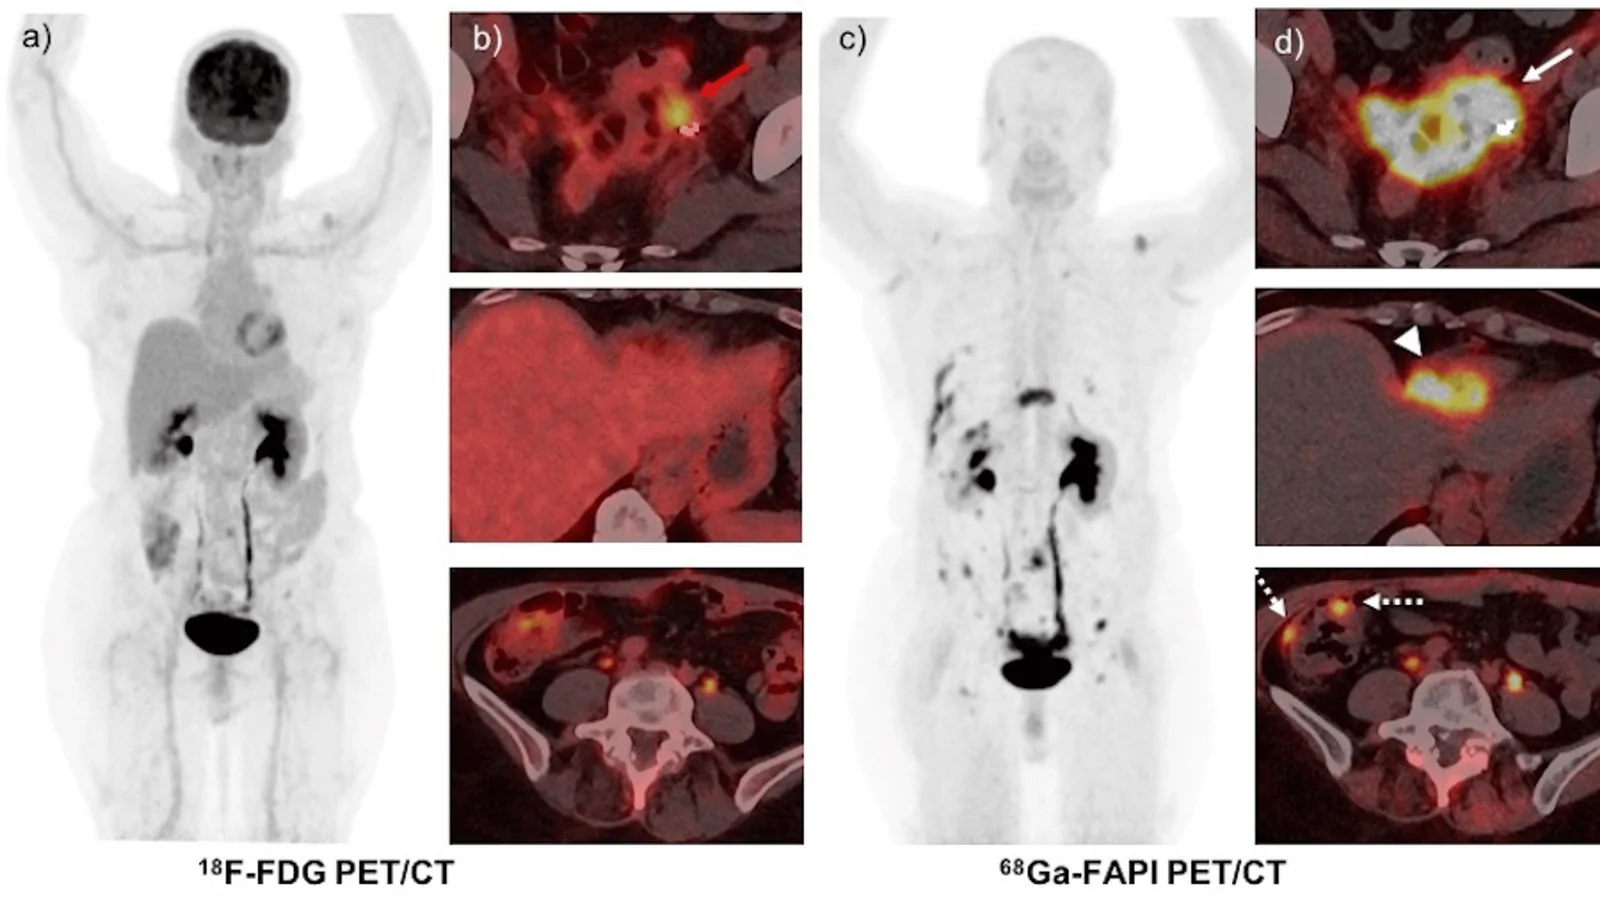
⁶⁸Ga-FAPI PET/CT in the evaluation of peritoneal disease (a) MIP and (b) fused ¹⁸F-FDG PET/CT images show a moderately avid infiltrative lesion (SUVmax 5.3, red arrow) at the vaginal stump abutting the rectum. (c) MIP and (d) fused ⁶⁸Ga-FAPI PET/CT images demonstrate a markedly avid lesion (SUVmax 15.1, white arrow) infiltrating the vaginal stump, rectosigmoid colon, and parametrial fat, with additional subdiaphragmatic, hepatic surface, bowel serosa (white dotted arrow), and diffuse peritoneal deposits. Findings highlight superior lesion detection and delineation with FAPI PET/CT, particularly for local recurrence and peritoneal carcinomatosis. ⁶⁸Ga: ⁶⁸Gallium; FAPI: fibroblast activation protein inhibitor; ¹⁸F-FDG: ¹⁸F-fluorodeoxyglucose; PET: positron emission tomography; CT: computed tomography; MIP: maximum intensity projection
